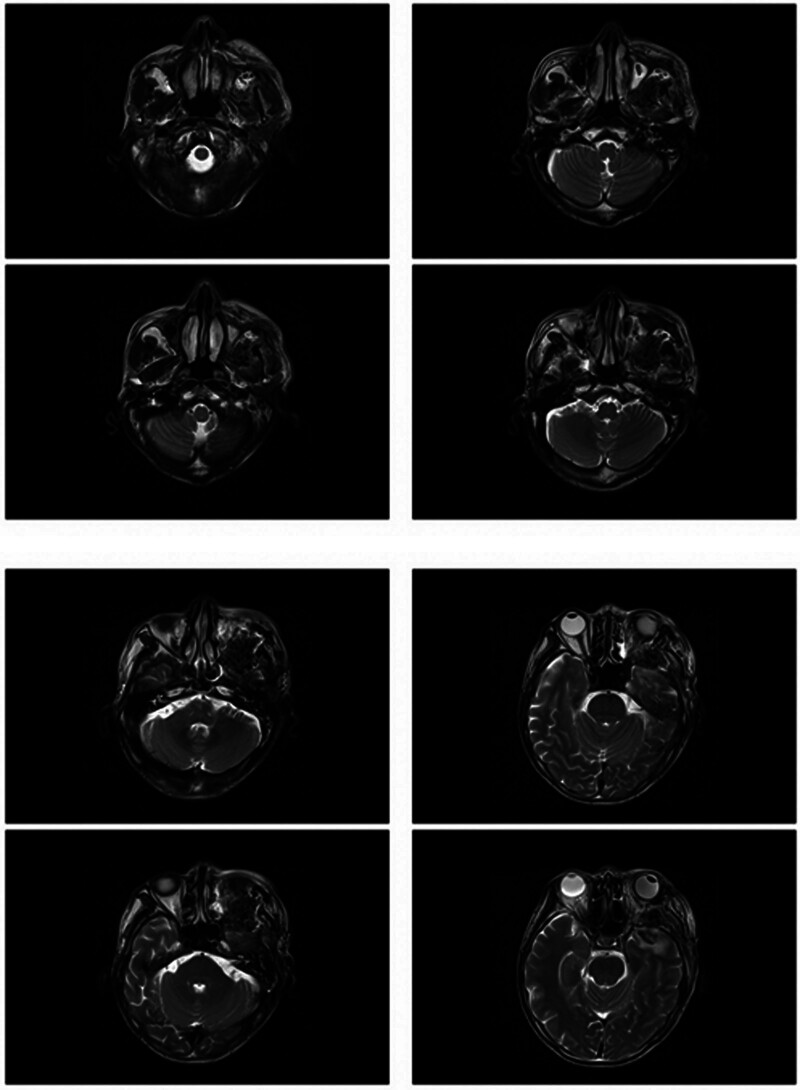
Patient postoperative MRI cross-sectional T2 phase. MRI = magnetic resonance imaging.
